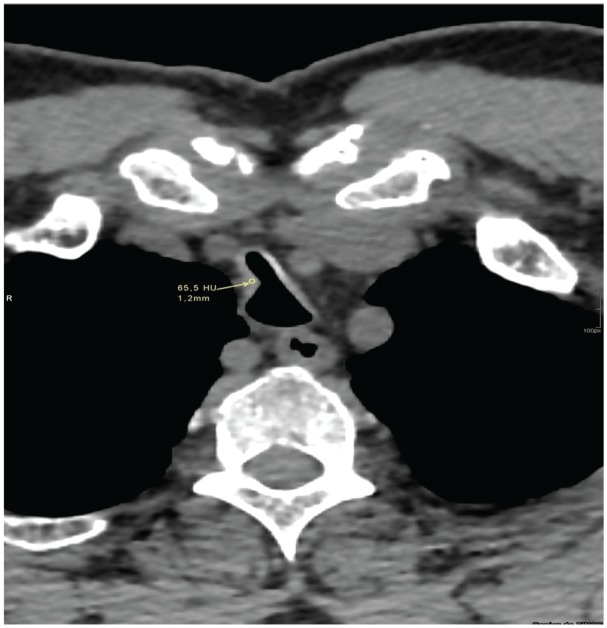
Thoracic axial computed tomography scan. Loss of normal tracheal morphology with soft tissue nodule (65 HU) on the right tracheal wall, without significant stenosis.
